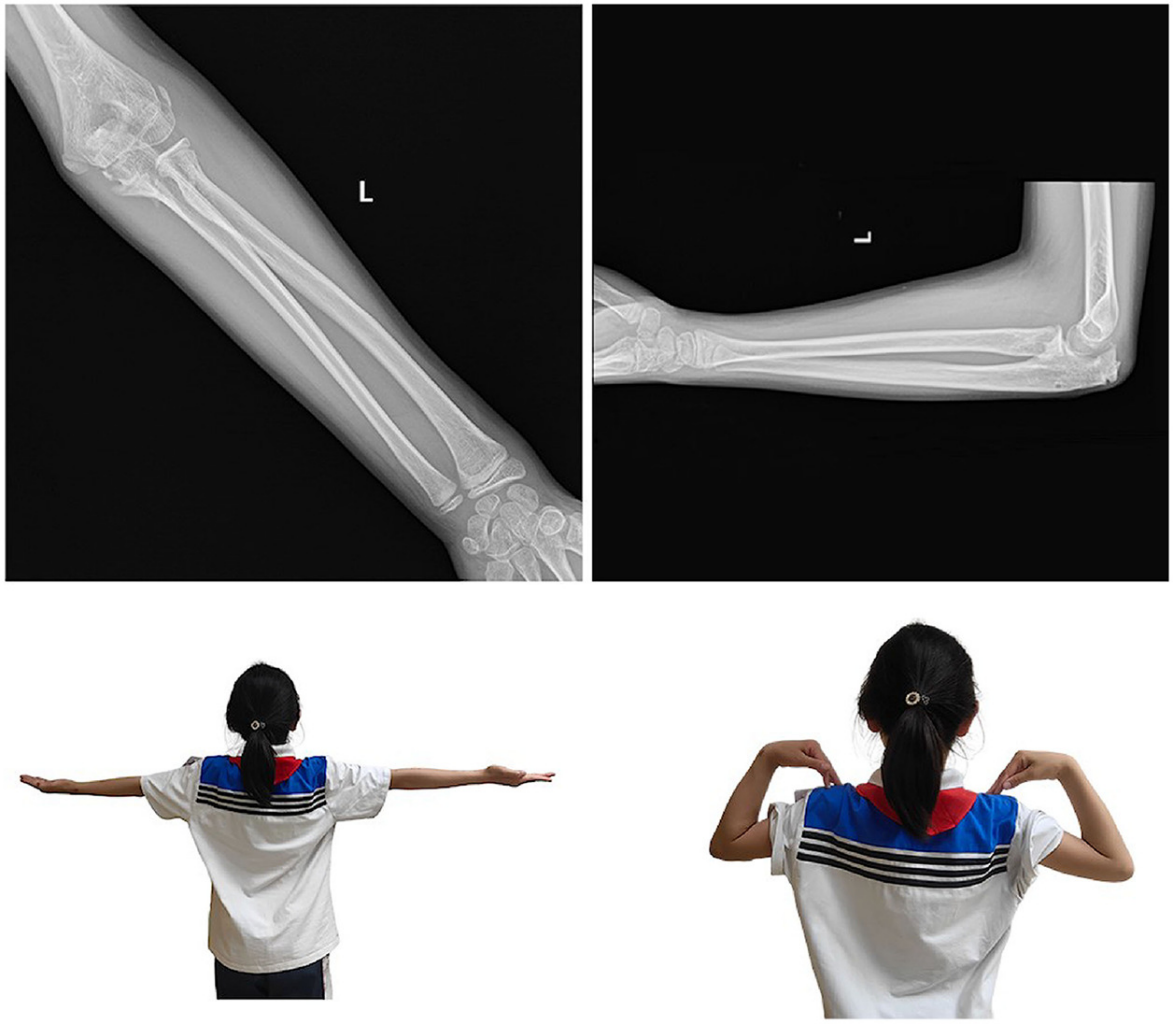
Postoperative x-rays at the one-year follow-up showed normal anatomical relationships of the elbow joints, and no functional difference was observed between the bilateral elbow joints.
